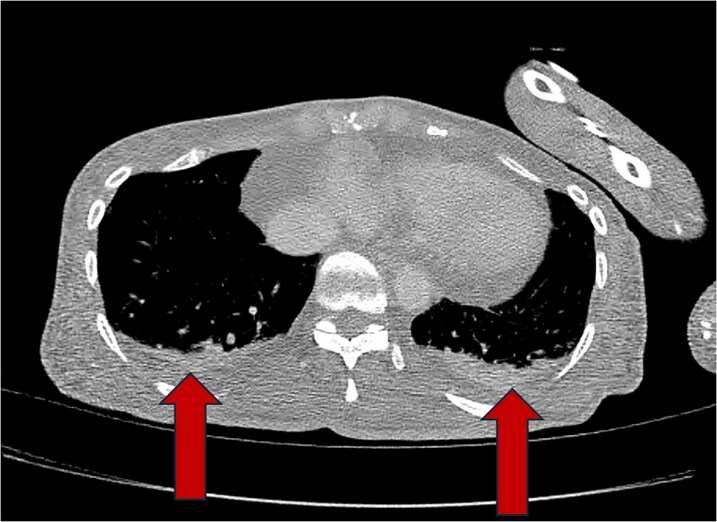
CT thorax showing bilateral pleural effusions and associated atelectasis/consolidation.
